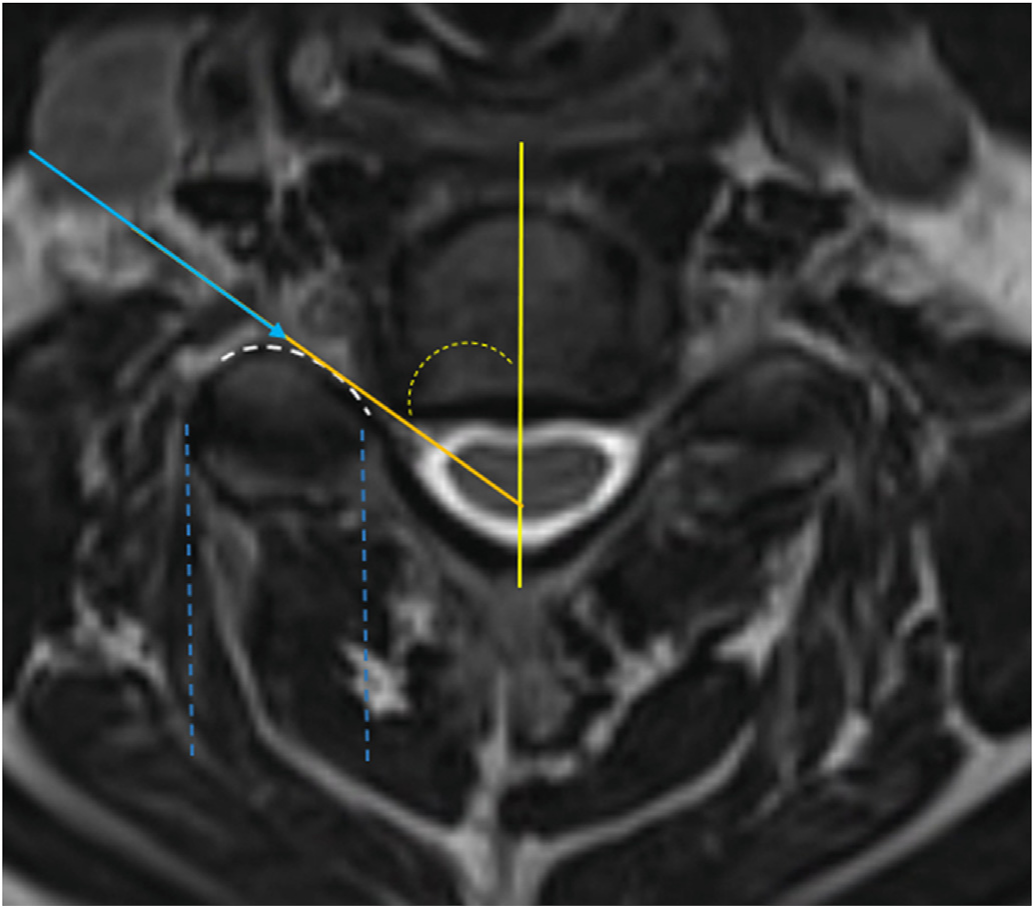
T2 axial MRI with curvilinear SAP morphology. Occasionally, the SAP is more of a curvilinear configuration rather than linear, as in Fig. 4. In such cases, the measurement procedure is similar to that demonstrated in Fig. 4 with a subtle modification; the line drawn along the ventral surface of the SAP (orange line) is tangential only to the medial half of the SAP which tends to be more linear. Note that a needle trajectory advanced at this given angle under fluoroscopy (solid blue arrow) is not likely to contact bone until the mid-portion of the articular pillar width (between dashed blue lines) when visualized on fluoroscopic AP view.
